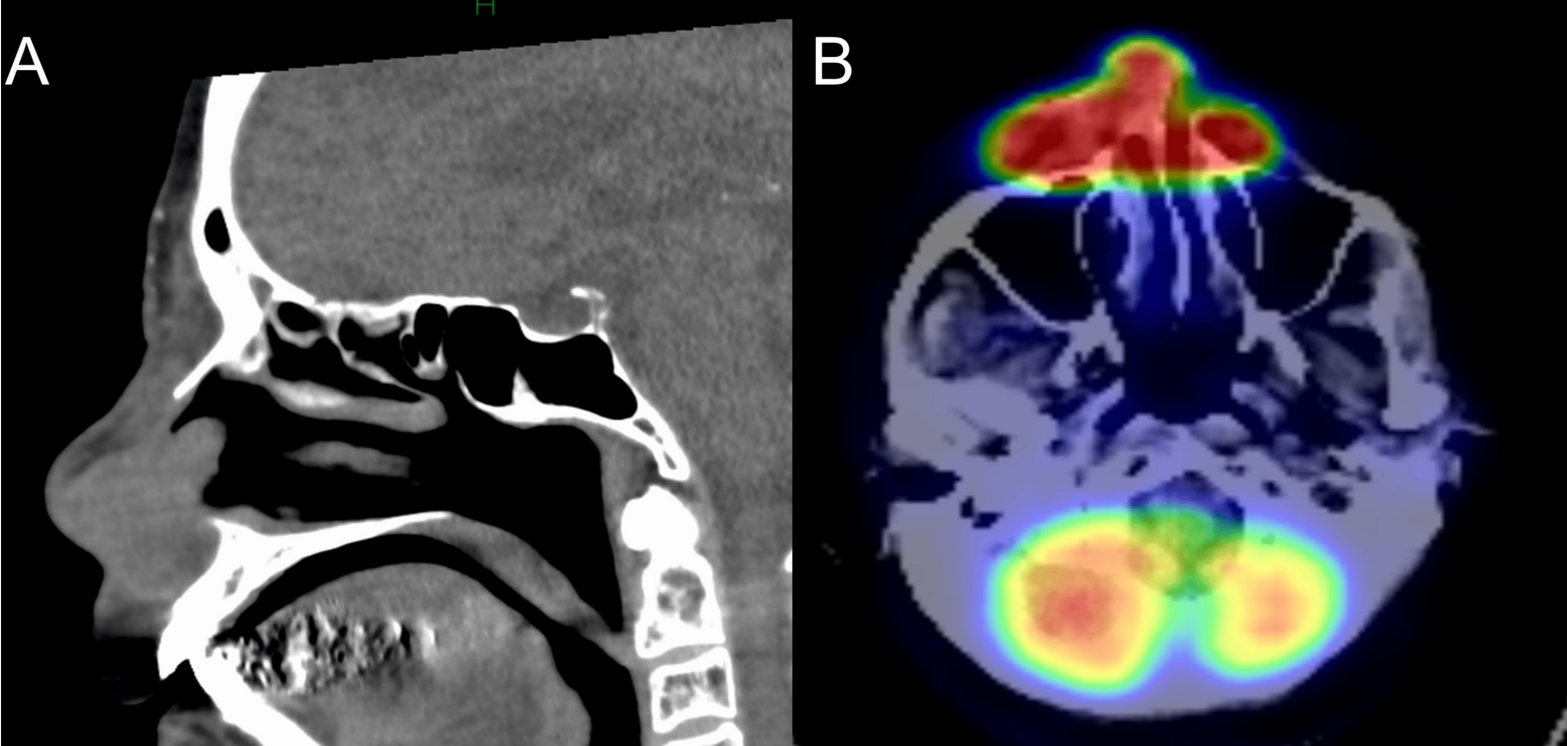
CT and PET-CT findings (A) CT scan showing skin thickening and increased density of the subcutaneous fat tissue in the nasal region. (B) PET-CT showing abnormal FDG uptake in the nasal and perinasal areas, with no evidence of systemic involvement.
